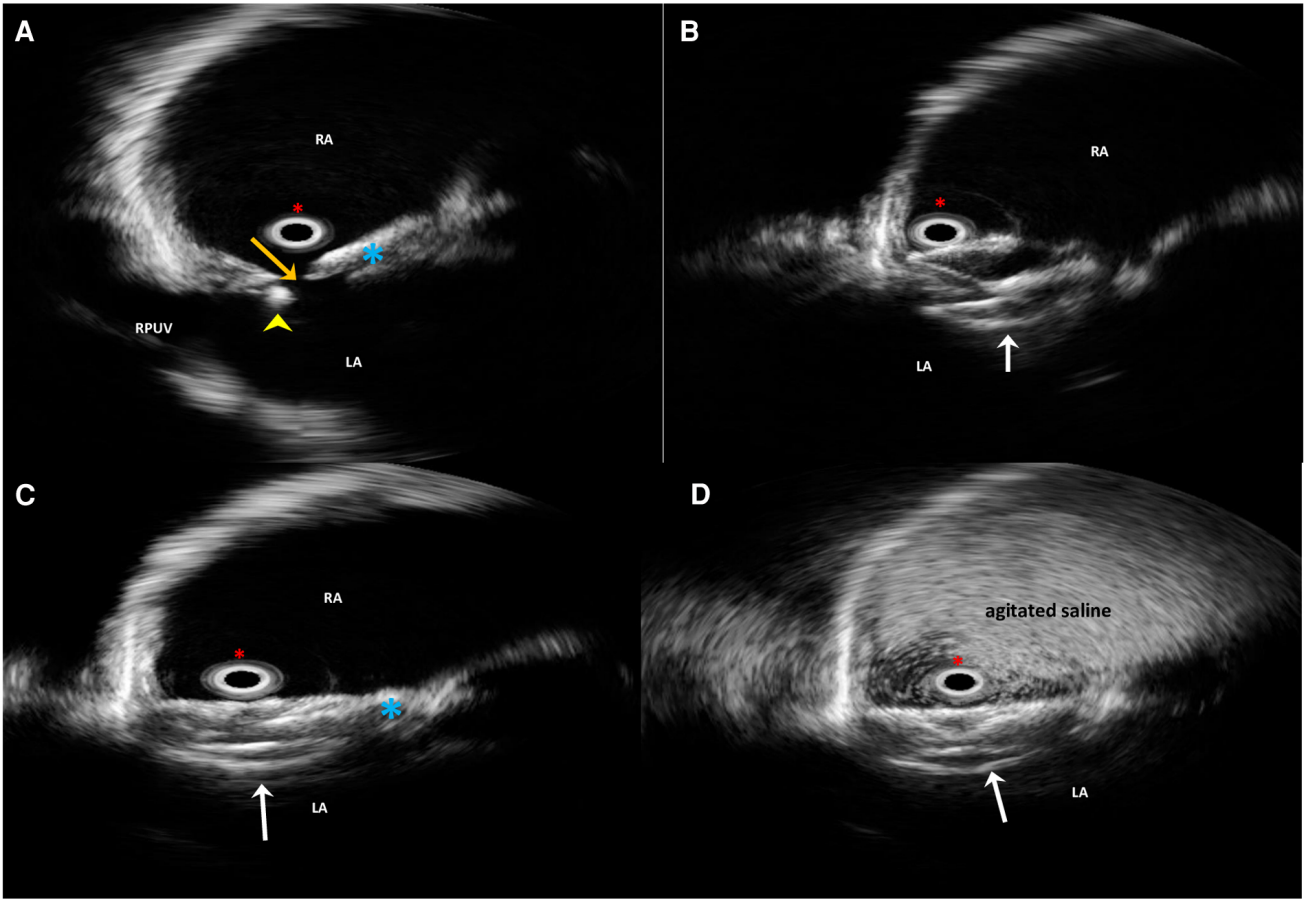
Long-axis four-chamber plane intraprocedural rotational intracardiac echocardiography by Ultra ICE (mechanical 9F/9 MHz 360° scan probe) procedural steps. The guidewire (yellow arrowhead) is across the residual septal defect (orange arrow) apart from the previously implanted device (light blue asterisk) (A). Successful implantation steps of the Flex II PFO 16/18-mm device (white arrows) (B,C). Contrast-enhanced Ultra ICE image confirming the abolition of the residual shunt (D). LA, left atrium; RA, right atrium; RUPV, right upper pulmonary vein. Red asterisk, the Ultra ICE-9F-9 MHz catheter located at the center of the image.
